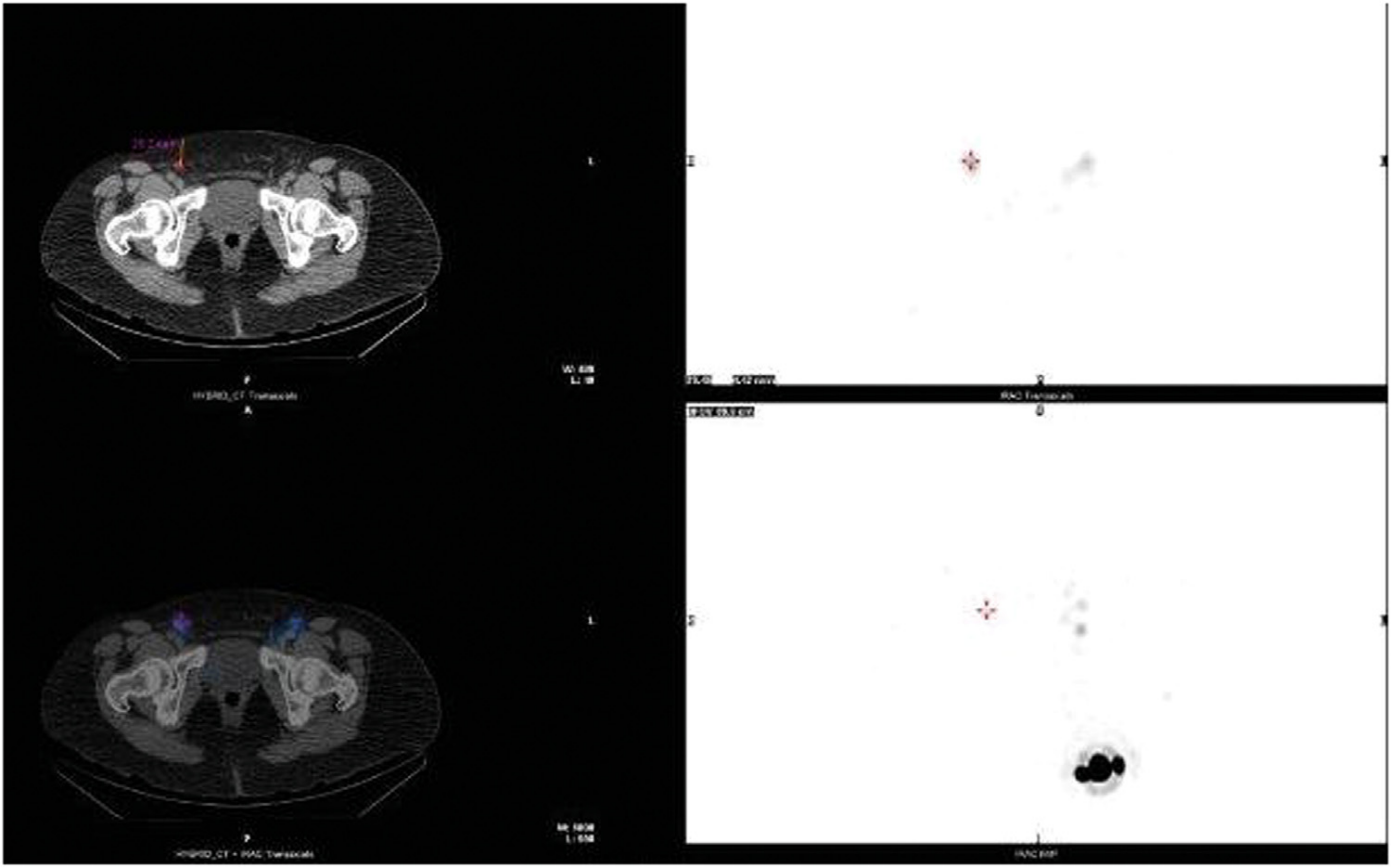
SPECT-CT confirming the presence of a contralateral sentinel lymph node.
